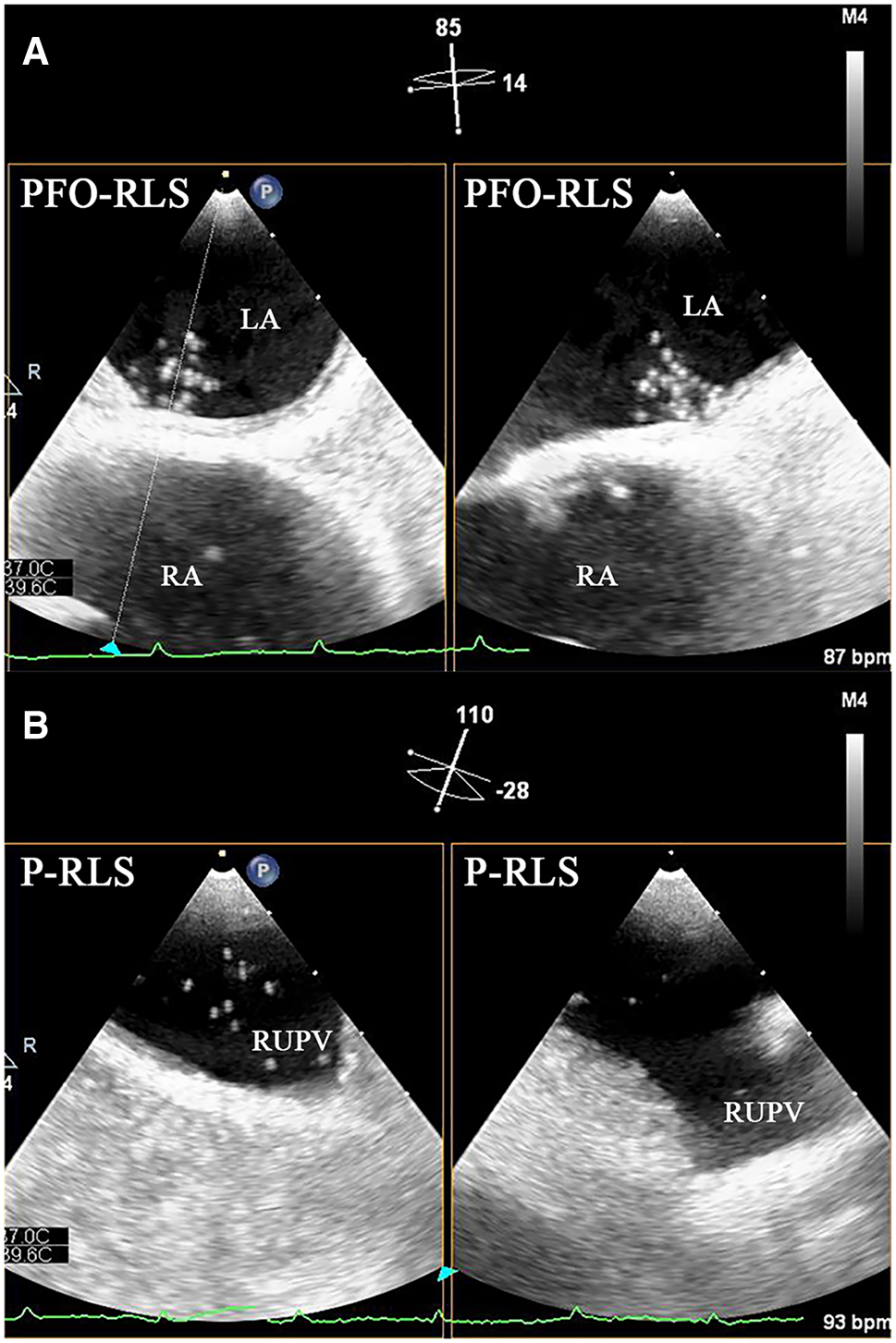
Biplane images of PFO-RLS and P-RLS under c-TEE. (A) PFO-RLS: microbubbles were observed to squeeze through the PFO. (B) P-RLS: microbubbles were observed to originate from the right superior pulmonary veins. PFO-RLS, patent foramen ovale right-to-left shunt; P-RLS, pulmonary right-to-left shunt; c-TEE, contrast transesophageal echocardiography; LA, left atrium; RA, right atrium; RUPV, right upper pulmonary vein.
